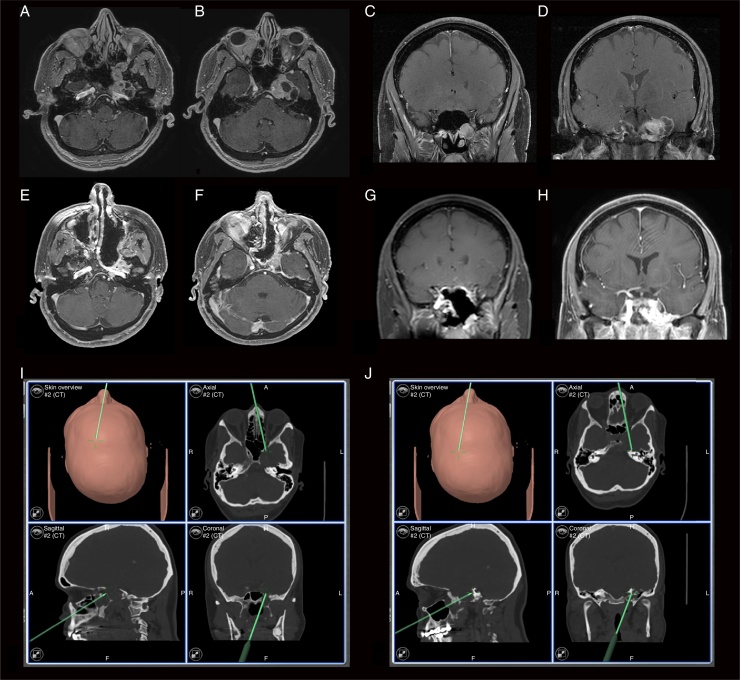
(A and B) Preoperative axial T1 MRI images with gadolinium; (C and D), preoperative coronal T1 MRI images with gadolinium; (E and F), postoperative axial T1 MRI images with gadolinium; (G and H), postoperative coronal T1 MRI images with gadolinium; (I), intraoperative neuronavigation image, showing the extension of the Vidian nerve canal (coronal); (J), intraoperative neuronavigation image showing posterior extent after lesion resection.
